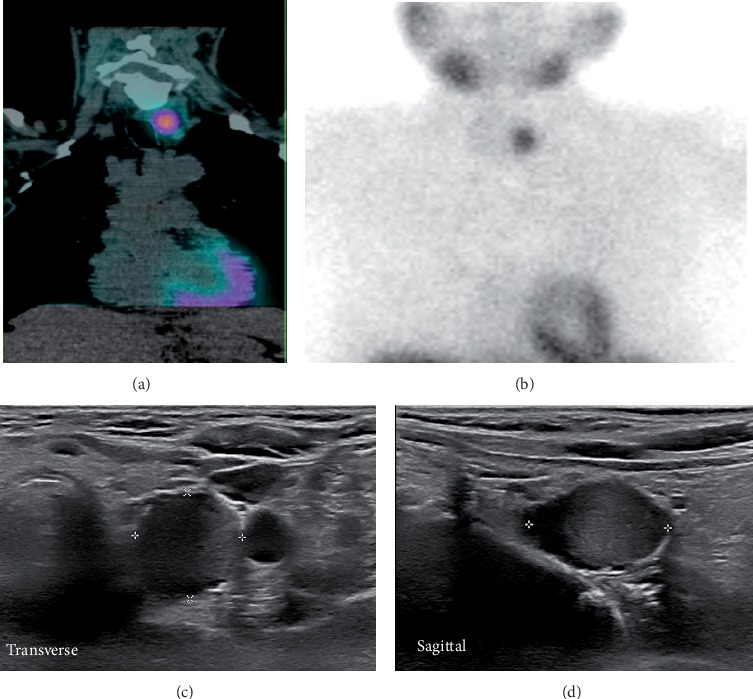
(a, b) 99mTc-sestamibi dual-phase with single-photon emission computed tomography/computed tomography fused images, showing uptake within middle to inferior left lobe of the thyroid. (b, c) Ultrasonograms revealing an oval-shaped hypoechoic solid nodule (1.40 × 1.39 × 1.92 cm) at the same area.
